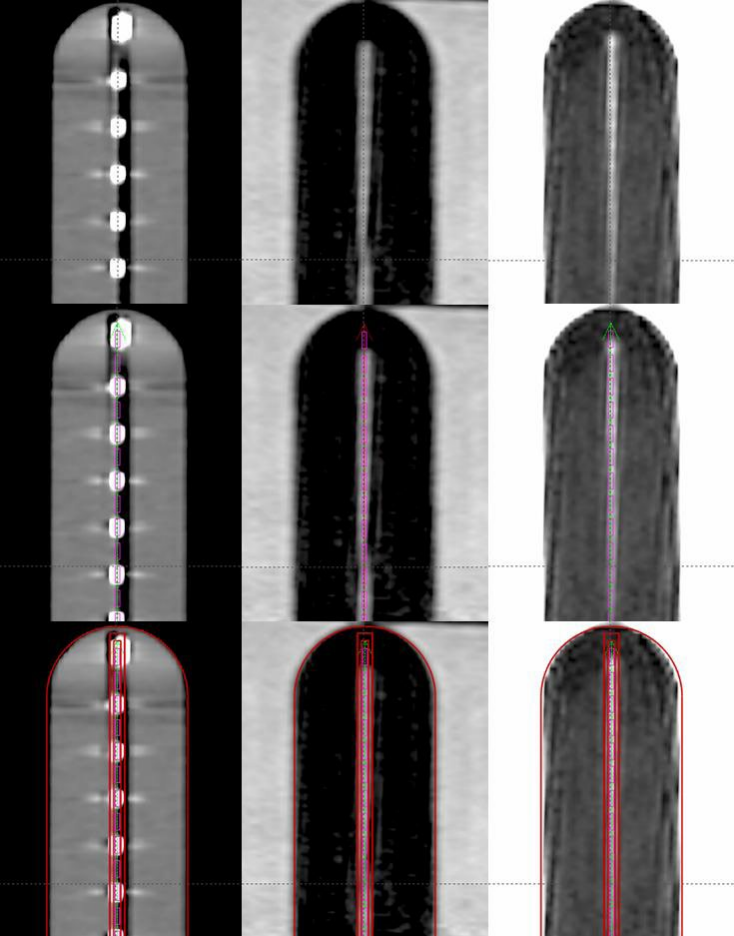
(Top row) Paracoronal view of the CT (left), T1W (middle), and T2W (right) images of the 30 mm standard vaginal cylinder with the appropriate marker in place. (Middle row) Applicator reconstruction using X‐ray markers on CT and overlaid on the MR images after the MR images were registered with the CT. (Bottom row) Applicator reconstruction using the solid applicator model aligned independently in each imaging modality.
